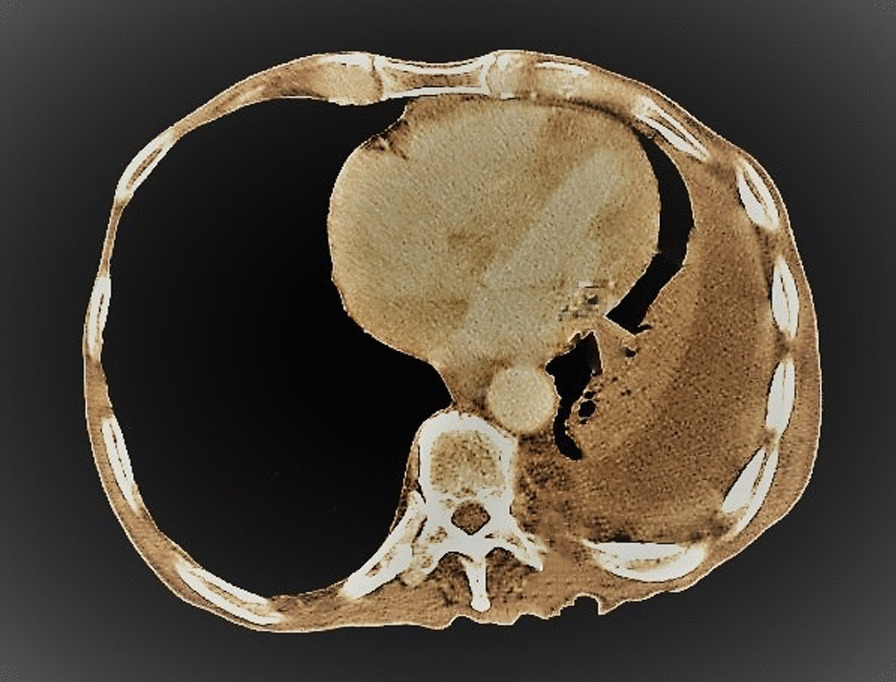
Computed tomography scan showing an intracardiac mass
